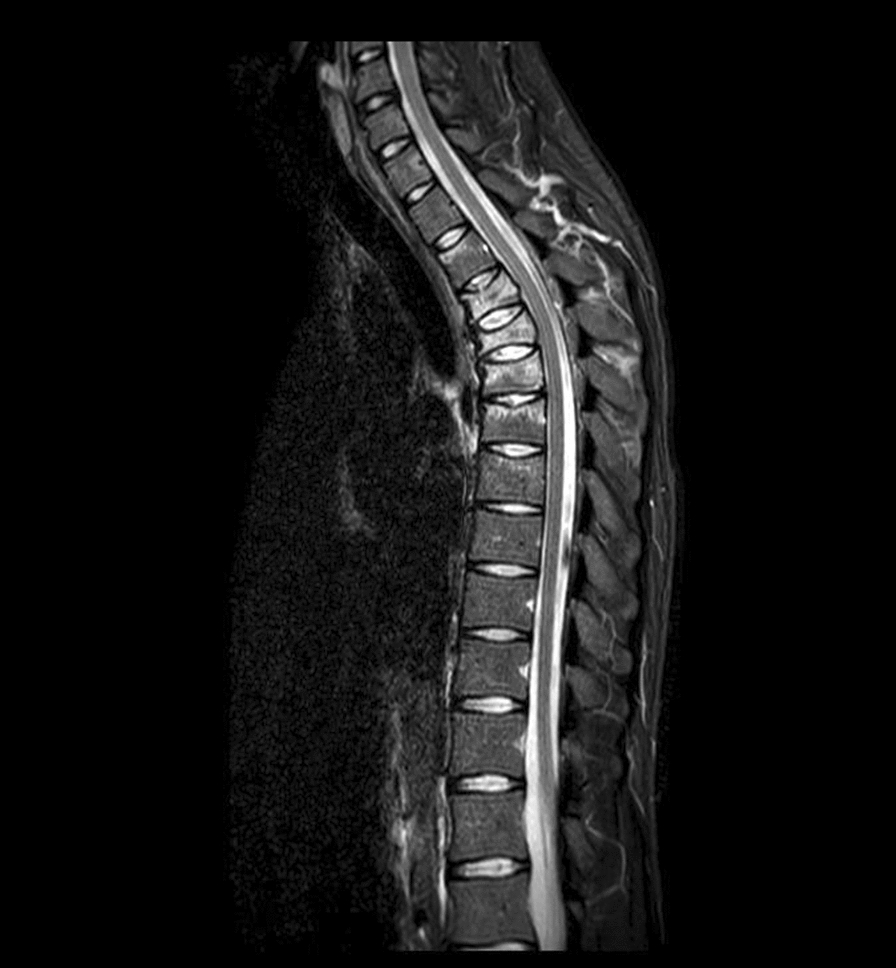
MRI scan confirming multiple compression fractures of thoracic vertebrae
